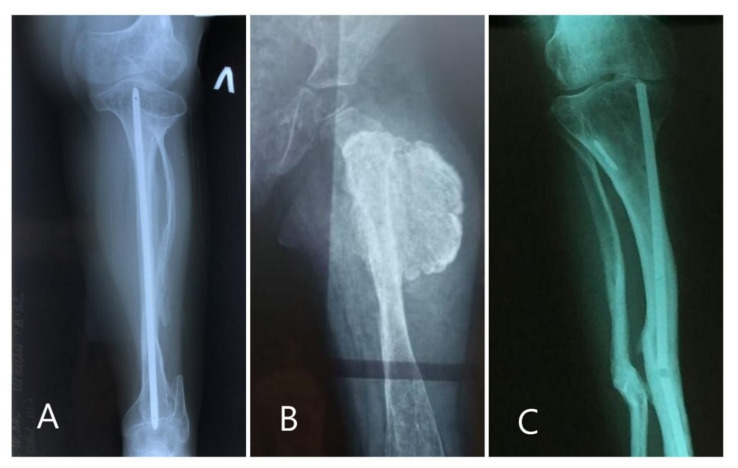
X-ray of patients 8 (A), 11 (B) and 12 (C). We see the absence of hypertrophic callus at the fracture site in patient 8, the presence of hypertrophic callus in patient 11 with the classic triad of signs and the absence of ossification of the interosseous membrane in patient 12.
